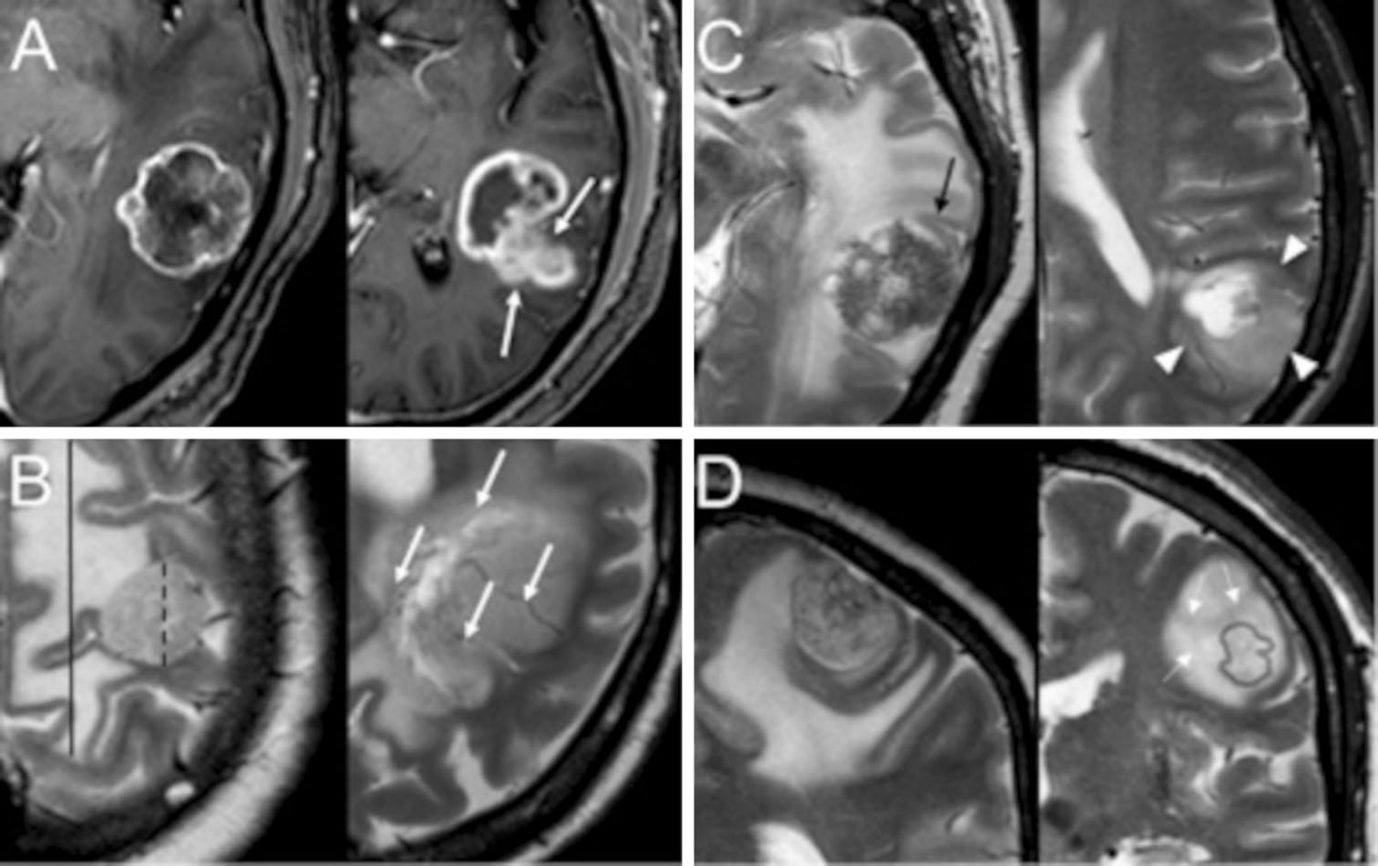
Analytic qualitative criteria used to differentiate single metastases (MET) from high-grade gliomas (GBM). a morphology and margins characteristics on post-contrast T1-weighted (T1w) axial images of a 66-year-old man with SBMMET from colon cancer (left), and in a 65-year-old man with GBM (right). SBM has an almost spherical shape and well-defined margins (arrows). In contrast, GBM exhibits an irregular shape, and areas with poorly defined margins. b Edema/lesion ratio and macroscopic vascularization on T2w axial images in a 56-year-old woman with SBM from breast cancer (left) and in a 71-year-old man with GBM (right). SBM has a high ratio between edema (continuous caliper) and lesion (dashed caliper). No prominent vessels coursing within the lesion are seen. In contrast, GBM has a relatively lower edema/lesion ratio and prominent intralesional vessels (arrows). c Lesion relationship with the cortex on T2w axial images, in a 66-year-old man with SBM (left, same as A) and in a 62-year-old man with GBM (right). SBM shows no thickening or definite signal change of the cortex in proximity of the lesion (arrow). However, GBM causes thickening and blurring of the cortex interface, suggesting infiltration (arrowheads). d T2-signal texture characteristics in the peri-enhancing region: coronal images of a 67-year-old woman with SBM from breast cancer (left) and a 75-year-old woman with GBM (right). Light gray outlines mark the corresponding enhancing nodules as seen on post-contrast T1w images. Whereas SBM exhibits a uniformly bright signal in the peri-enhancing region, suggesting simple vasogenic edema, GBM shows adjacent white matter signal inhomogeneity, with subtle hypointensities (arrowheads) suggesting tumor infiltration
